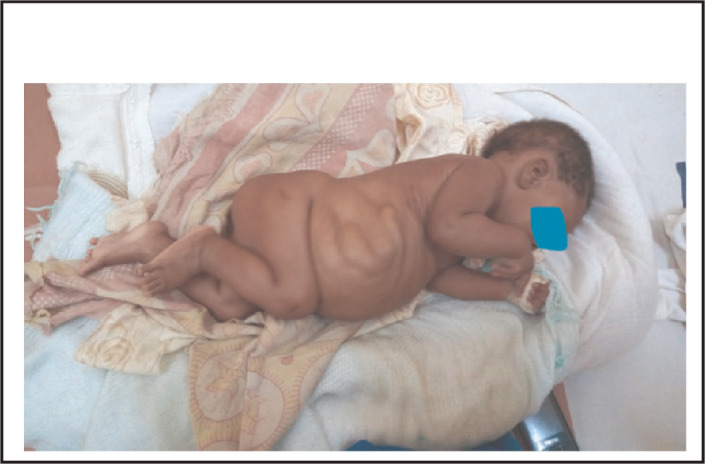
Laboratory investigations (Table 1) showed leukocytosis, predominantly of lymphocytes, microcytic hypochromic anaemia and almost normal creatinine. Chest x-ray showed opacities in the right upper lobe, suggestive of right upper lobe pneumonia, and enlarged costal-chondral junctions suggestive of rickets (Figure 5). Abdominal ultrasound showed bilateral hydronephrosis (Figure 6). After investigations, the child was treated as a case of severe pneumonia, improved after seven days of antibiotics (ampicillin and gentamicin) and was discharged with iron supplements and cod liver oil. His parents were also counselled about PBS and the care they can give him at home. He was given an appointment to come back for follow up after one month and referred to Bugando zonal referral hospital in Mwanza for surgical consultation and urology review, but he never got there. However, the patient was not seen till three months later when he was brought with complaints of diarrhoea, vomiting and fever for two weeks for which he been receiving - had been receiving treatment at Kabale dispensary without improvement. On arrival, the child was severely ill, restless, had sunken eyes and skin pinch went back slowly. His temperature was 39.3°C, with increased pulse rate and respiratory rate and peripheral capillary oxygen saturation (SpO_2_) of 92% on air. Other systems were normal. Laboratory investigations (Table 1) showed severe leukocytosis and granulocytosis with microcytic anaemia with low red blood cells (RBC). He was given intravenously administered antibiotics (Ceftriaxone) for treatment of suspected septicaemia, intravenous fluid to correct the hydration status, paediatric zinc, blood transfusion (140mls), and oxygen therapy. The child's condition continued to deteriorate and on the sixth day of admission he died.
